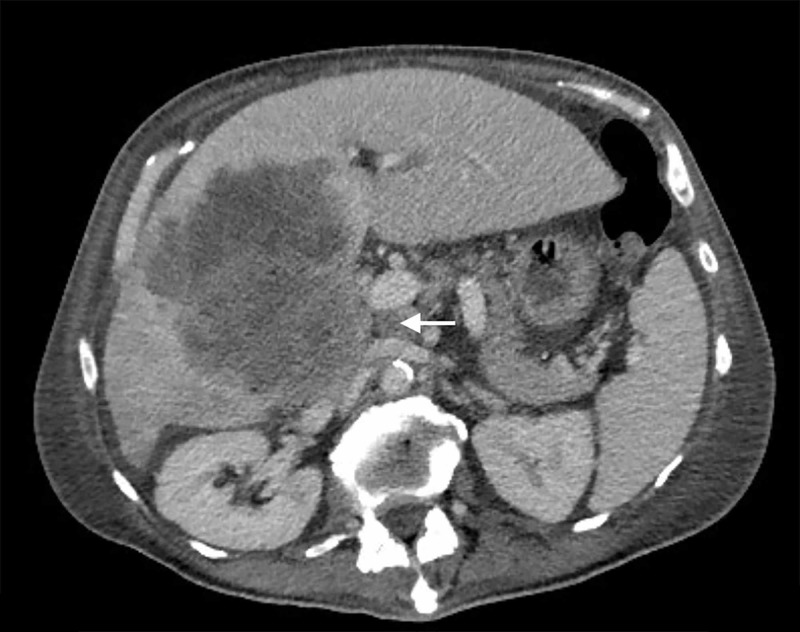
Coronal view of abdominal CT showing the mass compressing the suprarenal inferior vena cava at the point indicated by the arrow.
